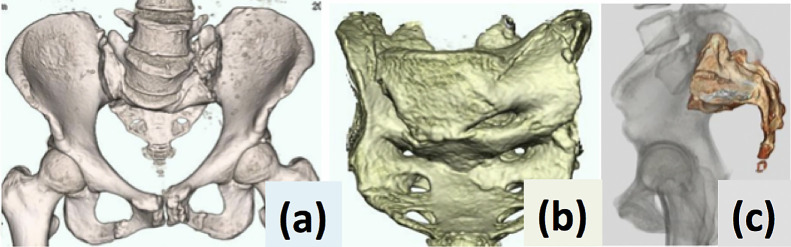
3D-CT of whole pelvis (a), anteroposterior view (b) and lateral view (c) of sacrum.
